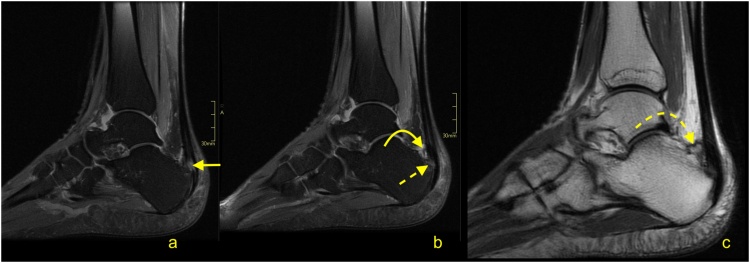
Status after the operation for Haglund's syndrome 6 months ago. Clinical improvement, reduction of pain, and swelling of Achilles tendon attachment. MRI revealed residual edema in the anterior part of the Achilles tendon insertion (straight arrow) with bone marrow edema in the calcaneus (dashed arrow). In the retrocalcaneal bursa, postoperative changes are visible as a slight inflammatory reaction (curved arrow) and adhesion (curved dashed arrow). A and b- PD- weighted with fat suppression, c- PD- weighted.
